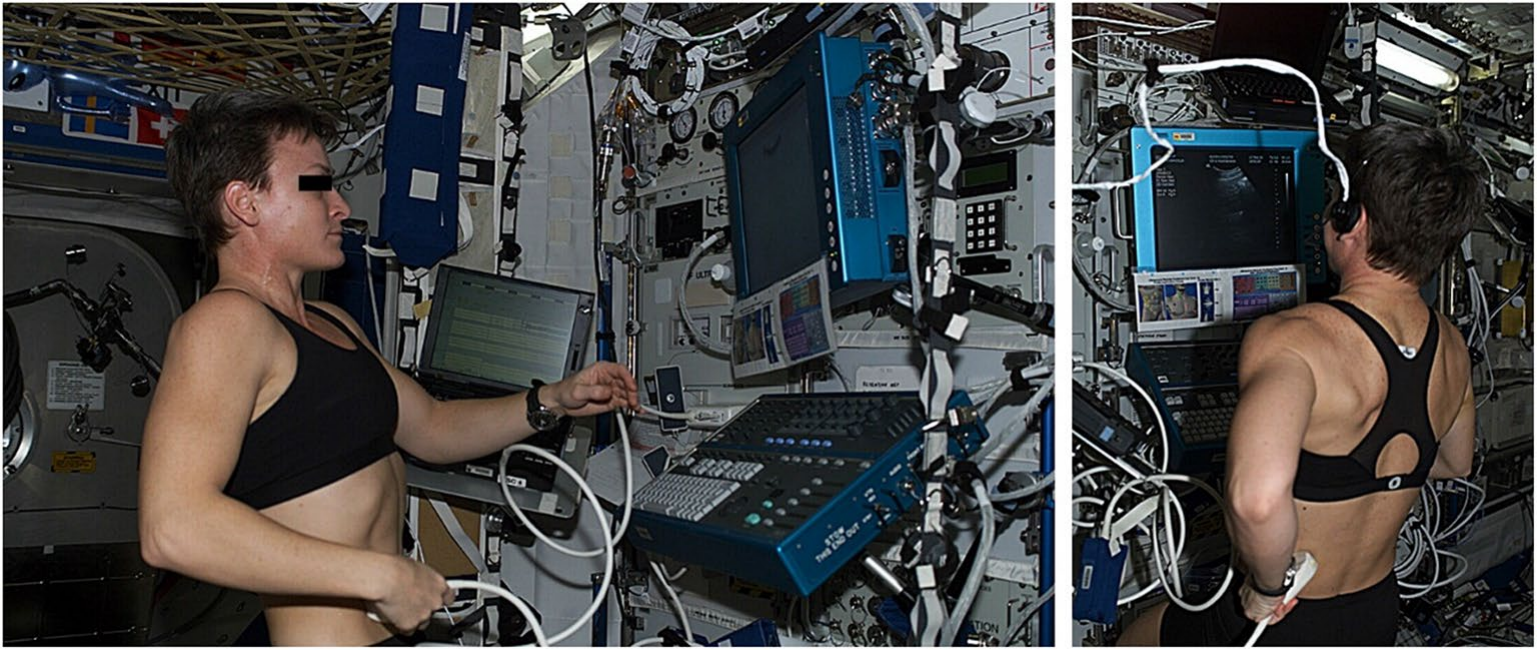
Crewmember aboard the ISS performing a guided renal ultrasound examination [31]
